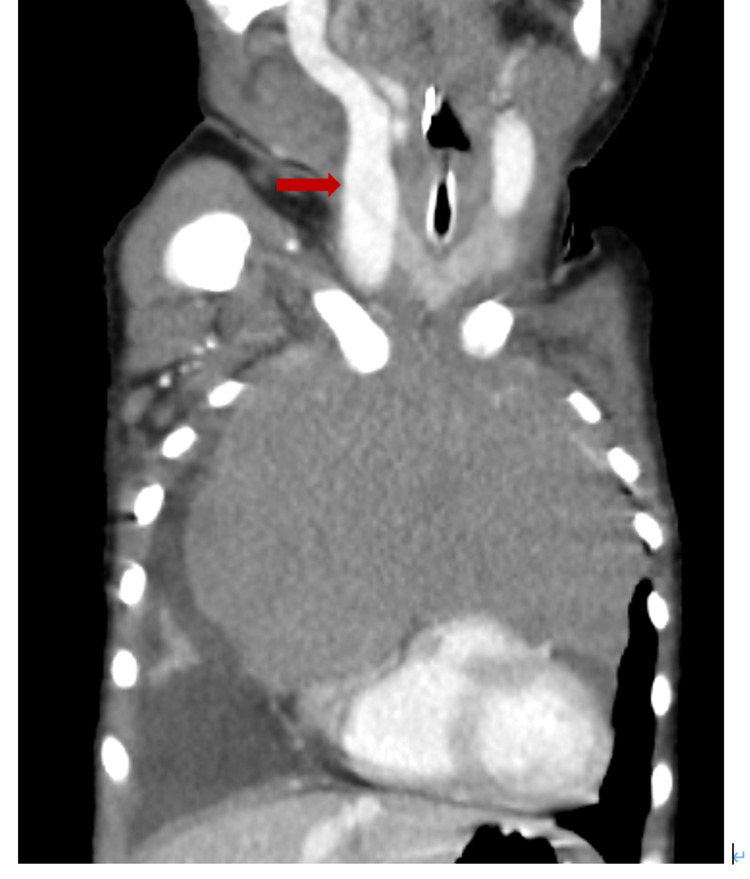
Contrast-enhanced CT Internal jugular veins are distended due to the compression of the superior vena cava.
